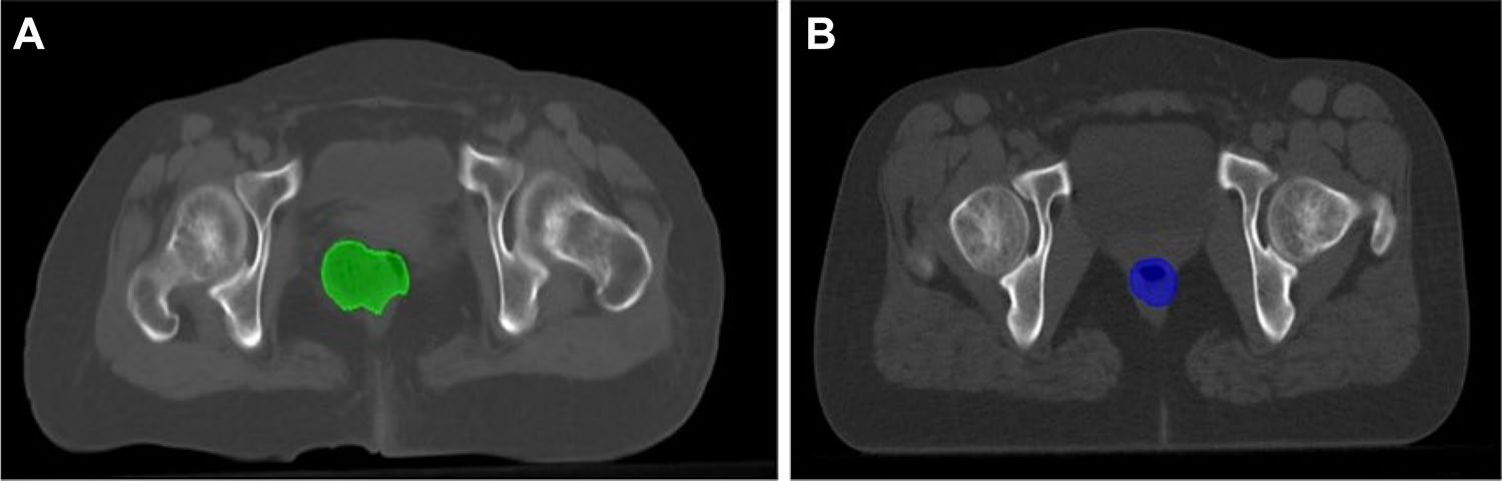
Representative trans-axial CT slices of two different patients showing the different rectum volume/location between two sets of CT images
